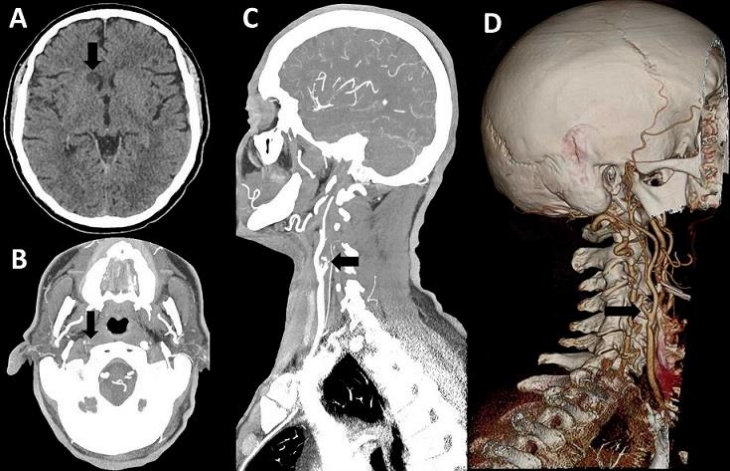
axial (A) view of cranial non-contrast CT scan showing hypodense round area in right medial lenticulo-striate artery territory, axial (B), sagittal (C), and volume-rendered (D) views of cranial CT angiography showing complete occlusion of right extracranial internal carotid artery
